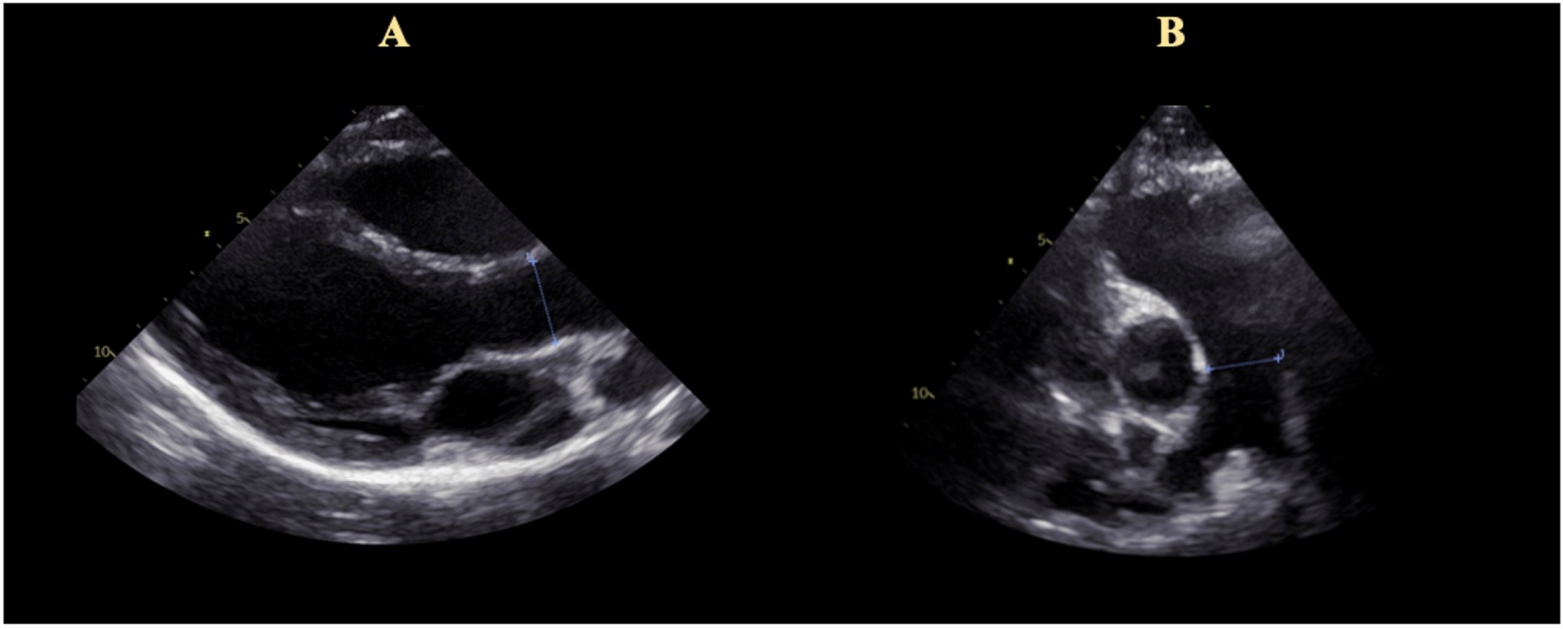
Calculation of the pulmonary artery to ascending aorta ratio (PA:A). Panel A: Parasternal long axis view with measurement of the ascending aorta. Panel B: Parasternal short axis view with visualization of the pulmonary trunk and the bifurcation. Measurement of the main pulmonary artery.
